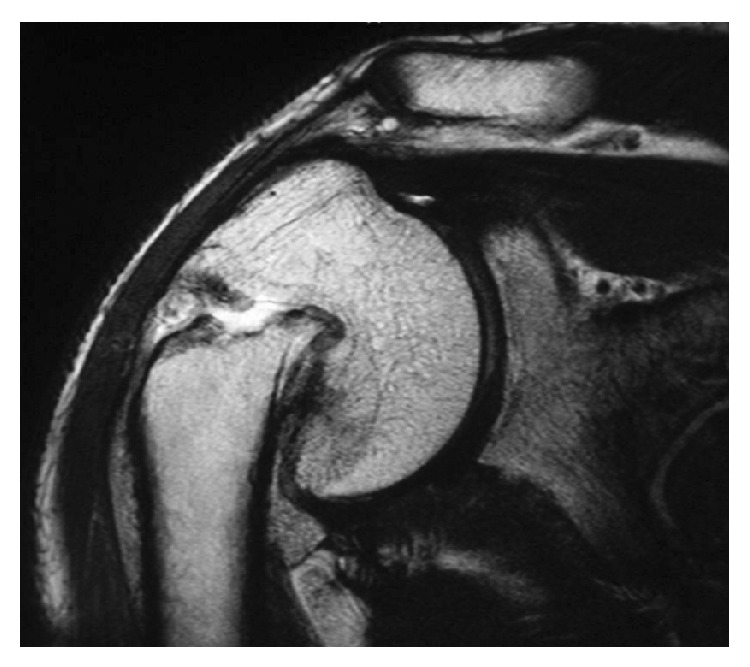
Preoperative T2-weighted MR image shows neither the rotator cuff tear nor the fluid collection in the subacromial bursa.
